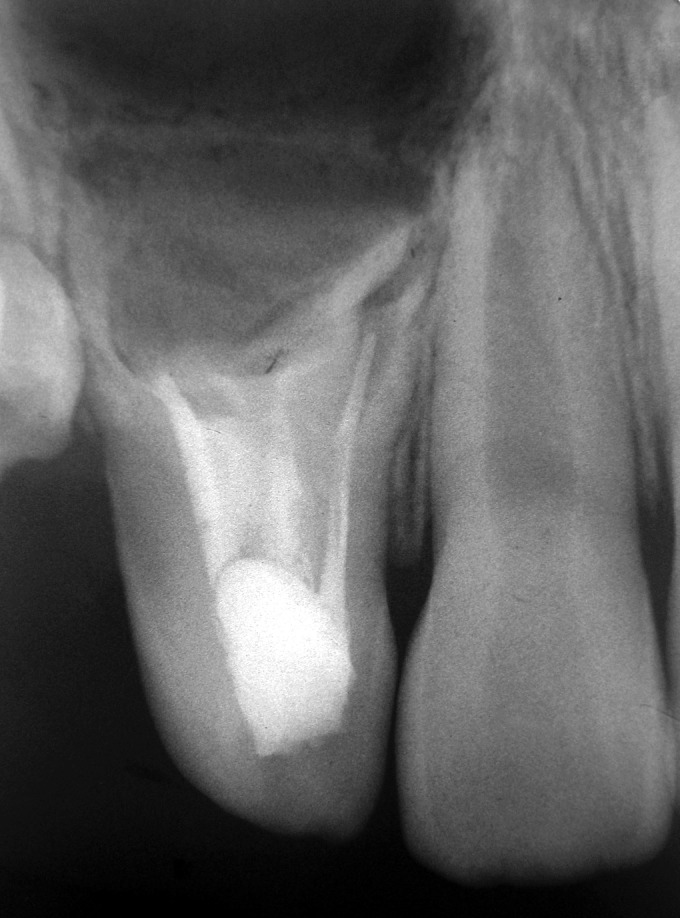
Follow-up radiograph after 1 year
